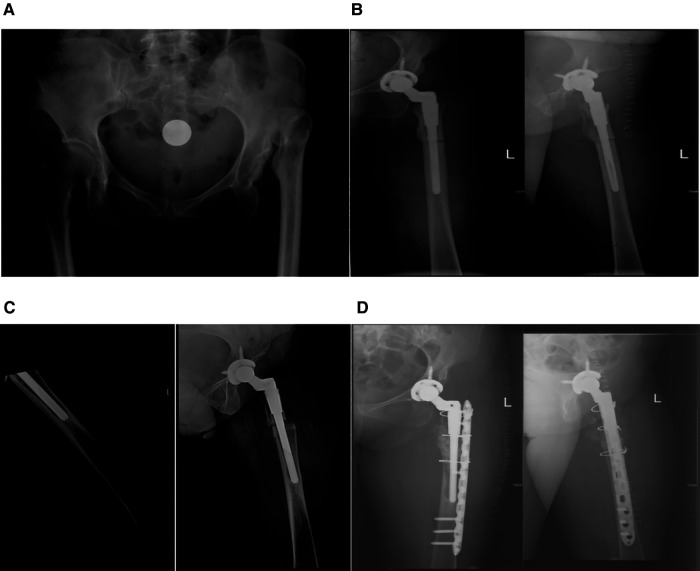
(A): anteroposterior pelvic x-ray with a diagnosis of left crowe IV DDH (left). (B): x-ray of initial subtrochanteric osteotomy with S-ROM prosthesis replacement. (C): After the patient fell, the distal end of the S-ROM prosthesis became loose, (D): x-ray of the left femur after revision of the left hip joint.
